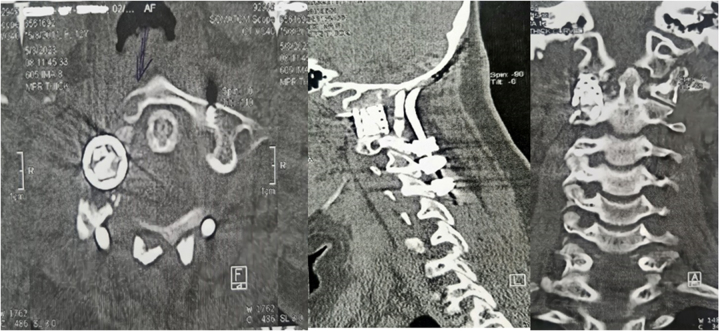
Cervical CT scan without contrast injection showing resection of the right C1 lateral mass ABC and reconstruction with titanium mesh cage. Right: anterior view; middle: lateral view; left: axial view.
